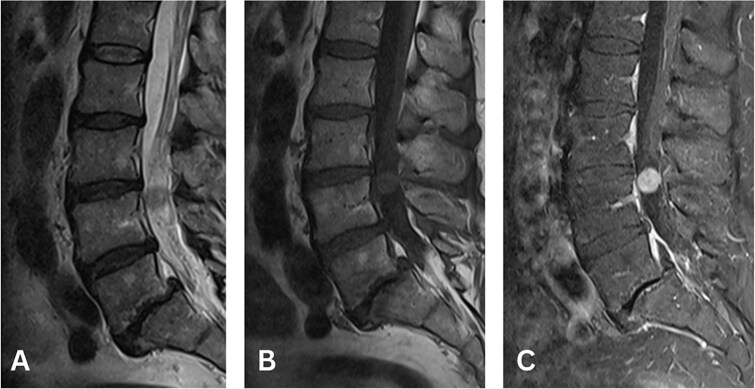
Magnetic resonance imaging (MRI) of the lumbar spine sagittal sequences revealing a round, intradural, extramedullary lesion at the level of the superior endplate of the L4 vertebral body which (A) on T2-weighted imaging (T2WI) demonstrates heterogeneous iso to hyperintense signal, (B) on T1WI demonstrates isointense signal, and (C) on post-contrast T1WI with fat suppression demonstrates homogenous diffuse enhancement.
